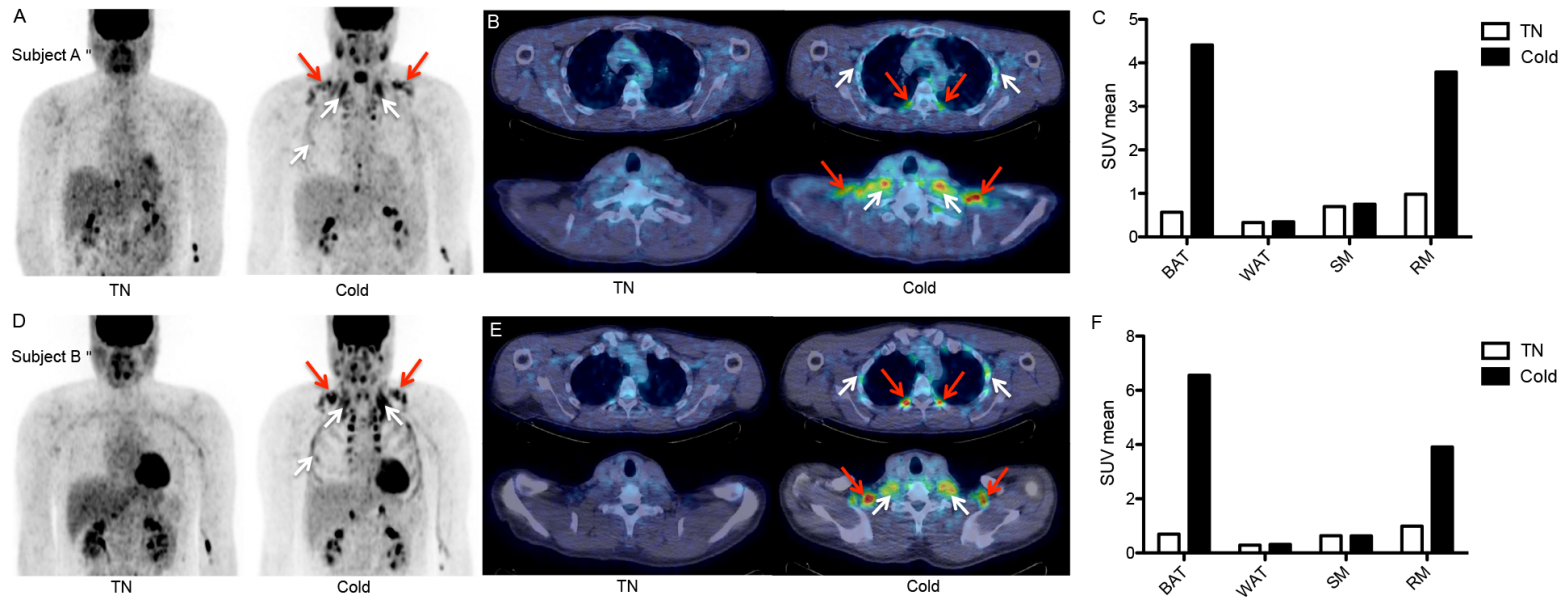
Brown adipose tissue and respiratory muscle activity during the thermoneutral and cold exposure experiment. A, D ) PET images during thermoneutral (left) and cold (right) conditions showing [^18^F]FDG-uptake e in brown adipose tissue (BAT; red arrows) and respiratory muscles (RM; white arrows). B, E) Transaxial slices of subject A (5 mm thick) of thoracic area (upper) and supraclavicular area (lower) demonstrating BAT activity (red arrows) and RM activity (white arrows). C, F) [^18^F]FDG-uptake (SUV_mean_) in BAT, white adipose tissue (WAT), skeletal muscle (SM), and respiratory muscles (RM) during thermoneutral and cold conditions.
